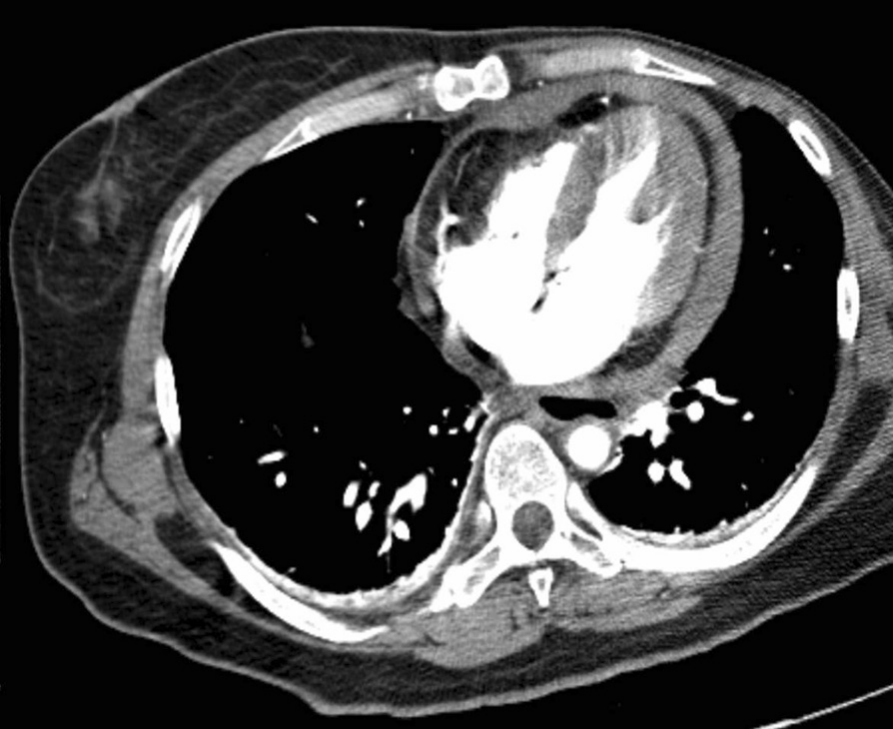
A computed tomogram demonstrated a pericardial fluid collection around the heart.
